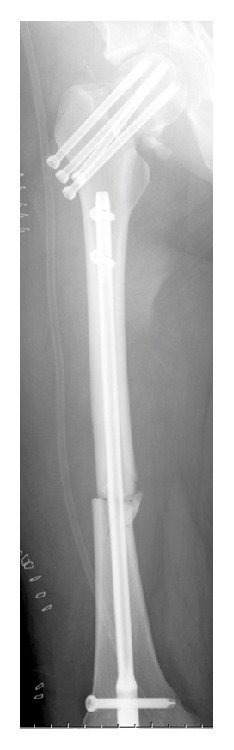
A postoperative radiograph of the femur. Retrograde intramedullary nailing for the right femoral shaft fracture and multiple cannulated cancellous screw fixation for the femoral neck fracture are performed.
